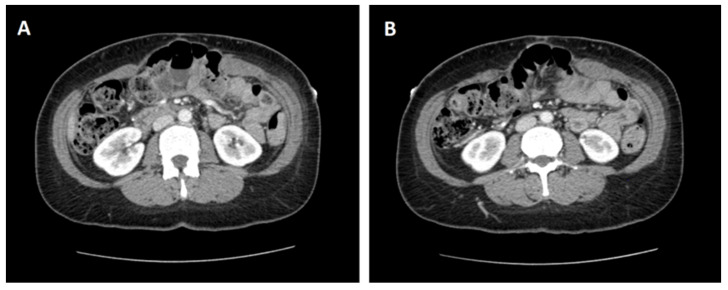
Transverse computed tomography (CT) slices at the level of L2 (A) and L3 (B) indicating a large ventral incisional hernia containing loops of the small and large bowel.
